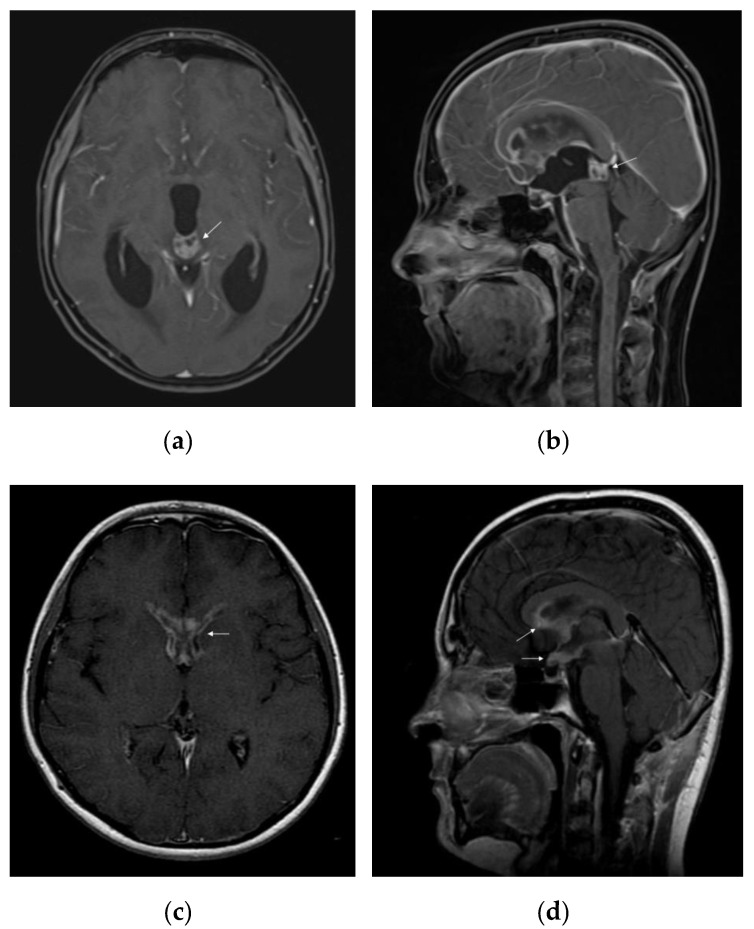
MRI features of intracranial germinoma in a teenage patient: (a) pineal germinoma: heterogeneous contrast enhancement on axial gadolinium-enhanced T1-weighted image, with a tendency to cardioid shape; (b) pineal germinoma: heterogeneous contrast enhancement on sagittal gadolinium-enhanced T1-weighted image; (c) postoperative disseminated disease: bilateral nodular enhancement of anterior horns of the lateral ventricles on axial gadolinium-enhanced T1-weighted image; (d) postoperative disseminated disease: nodular enhancement of the hypothalamus, optic chiasm, mammillary bodies, and corpus callosum on sagittal gadolinium-enhanced T1-weighted image.
